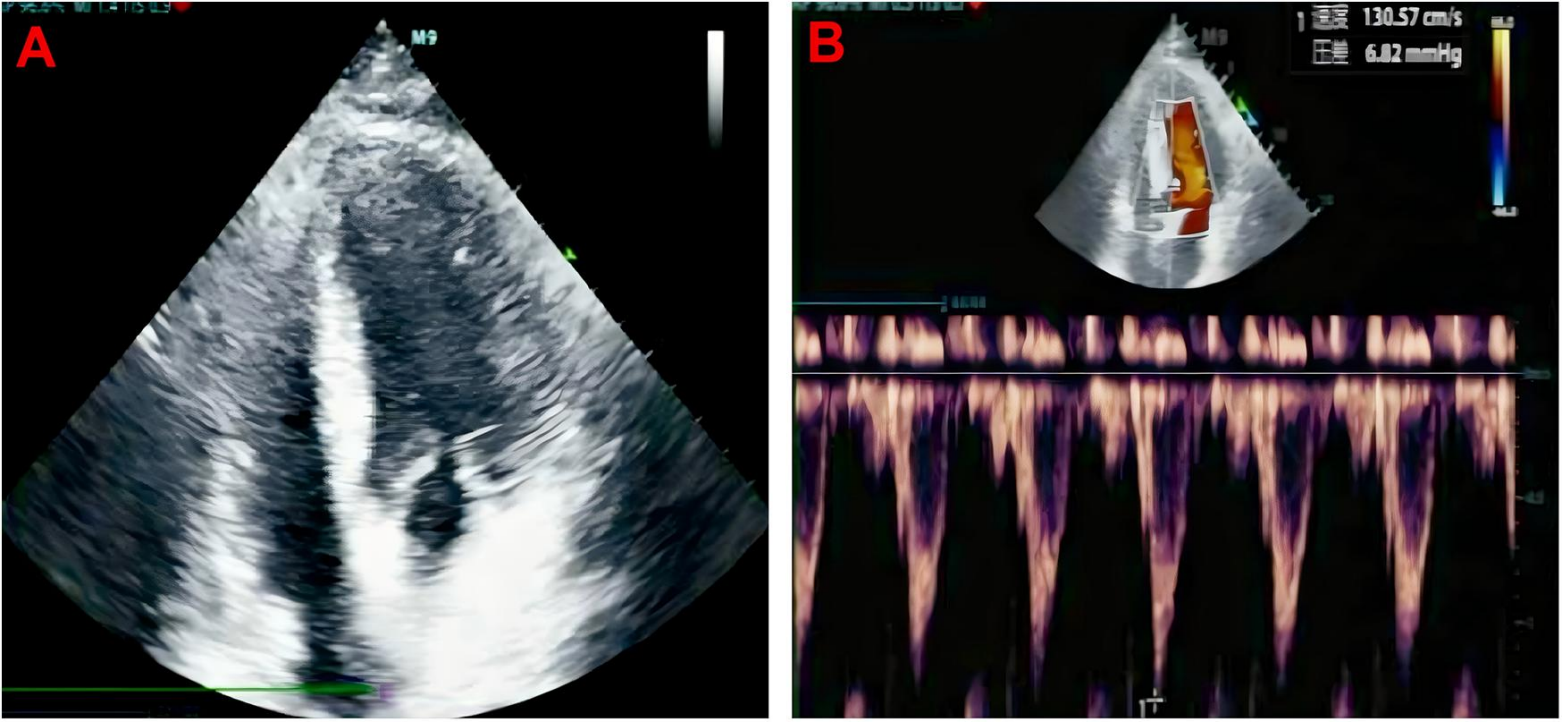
Normal apical four chamber echocardiography (A), normal four chamber aortic valve blood flow spectrum (B). Cardiac ultrasound did not show any structural abnormalities in the heart.
